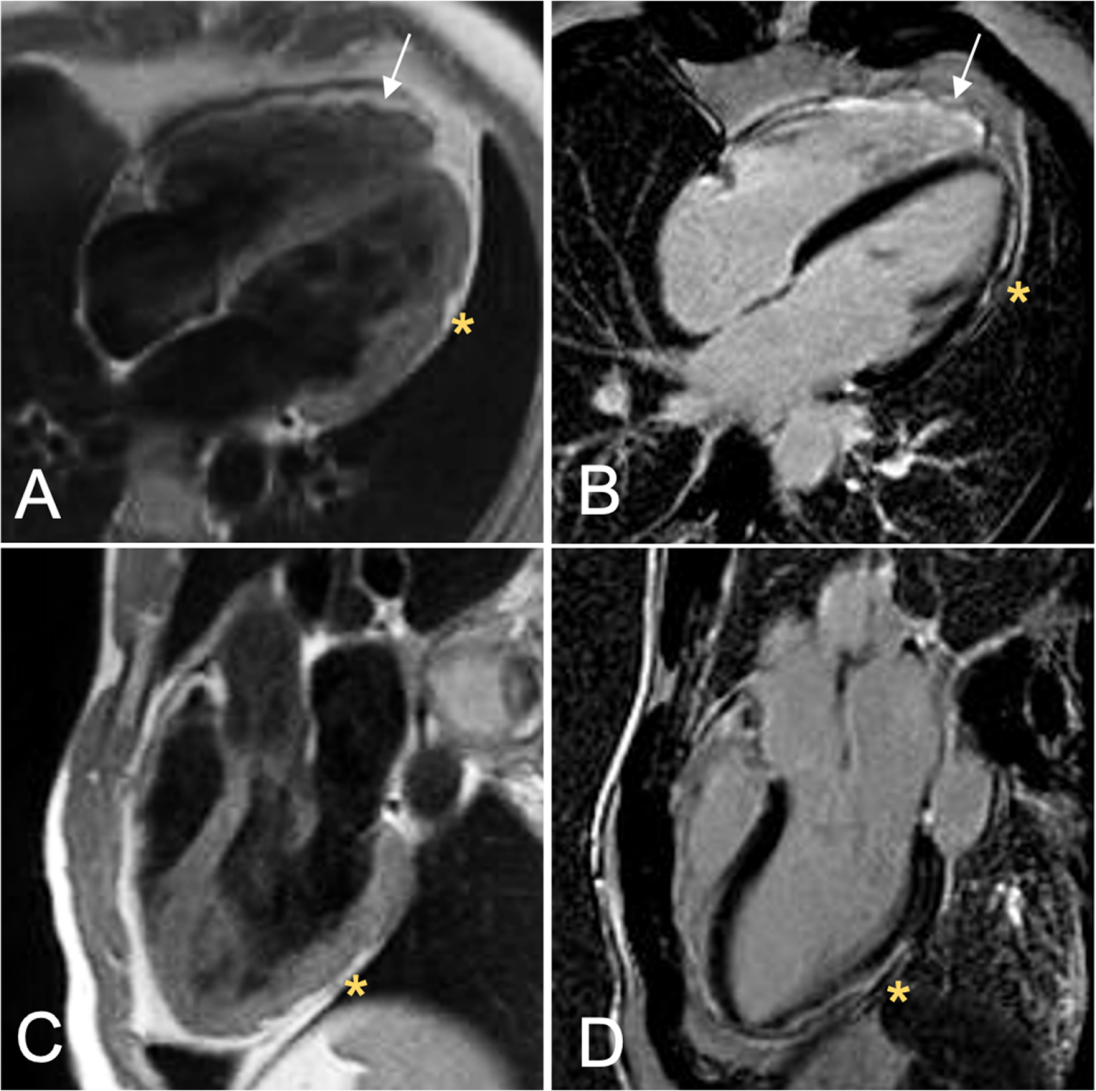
CMR in a patient with biventricular ACM demonstrating the fibro-fatty infiltration in the RV free wall (A, B; arrow) and the concomitant involvement of the LV lateral wall (A–D, asterisks). In details, T1-weighted (A) and post-contrast images (B) four chamber views showed the fibro-fatty infiltration of the LV antero-lateral wall (A, B; yellow asterisks) and of the apical RV wall (A, B; white arrows) and T1-weigheted (C) and post-contrast sequences (D) three chamber views showed that also the LV infero-lateral wall (C, D; yellow asterisks) was involved by the disease. ACM, arrhythmogenic cardiomyopathy; LV, left ventricle; RV, right ventricle
